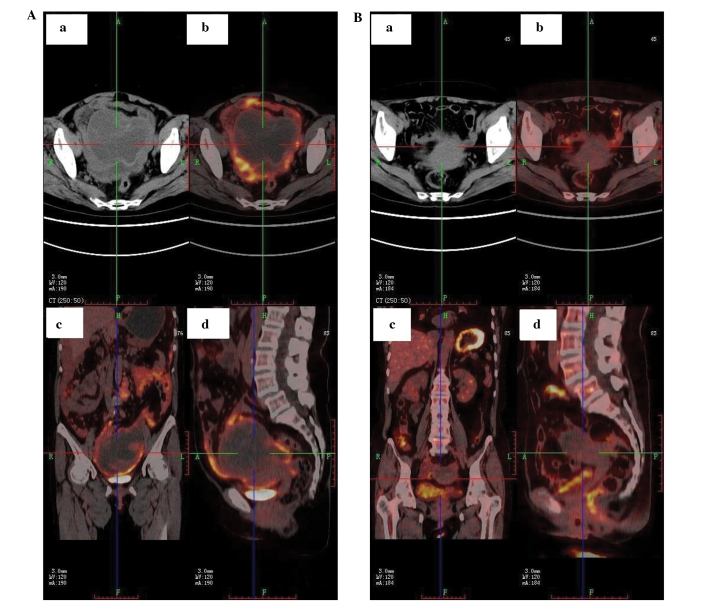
(A) Imaging of the patient prior to treatment. (Aa) Transverse CT and (Ab) transverse, (Ac) coronary and (Ad) sagittal PET scans of the patient, revealing the presence of metastatic cancer. (B) Imaging of the patient following treatment. (Ba) Transverse CT and (Bb) transeverse, (Bc) coronary and (Bd) sagittal PET scans of the patient, showing the disappearance of the tumor in the pelvic cavity. CT, computed tomography; PET, positron emission tomography.
